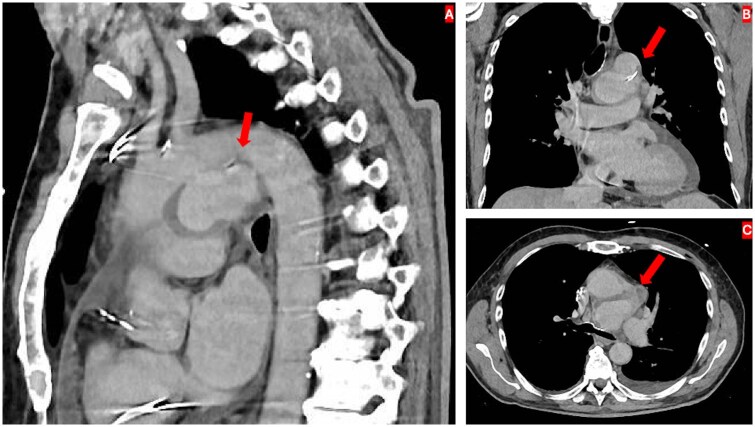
Sagittal (A), Coronal (B), and Transverse (C) Sections of Preoperative Contrast-Enhanced Computed Tomography Showing a Distal Arch Pseudoaneurysm from a Penetrating Aortic Ulcer Measuring 4.5 × 7.2 × 3.7 cm
